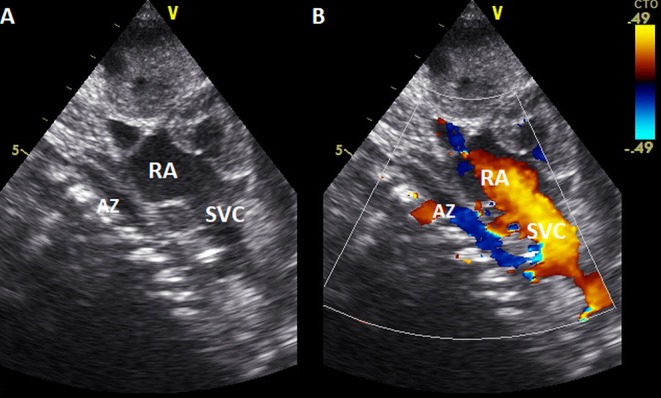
Echocardiography (2D and color Doppler, A, B, respectively) subcostal view, showing the ascending of azygos vein in the posterior mediastinum before entering in the superior vena cava. AZ, Azygos vein; RA, right atrium; SVC, superior vena cava.
